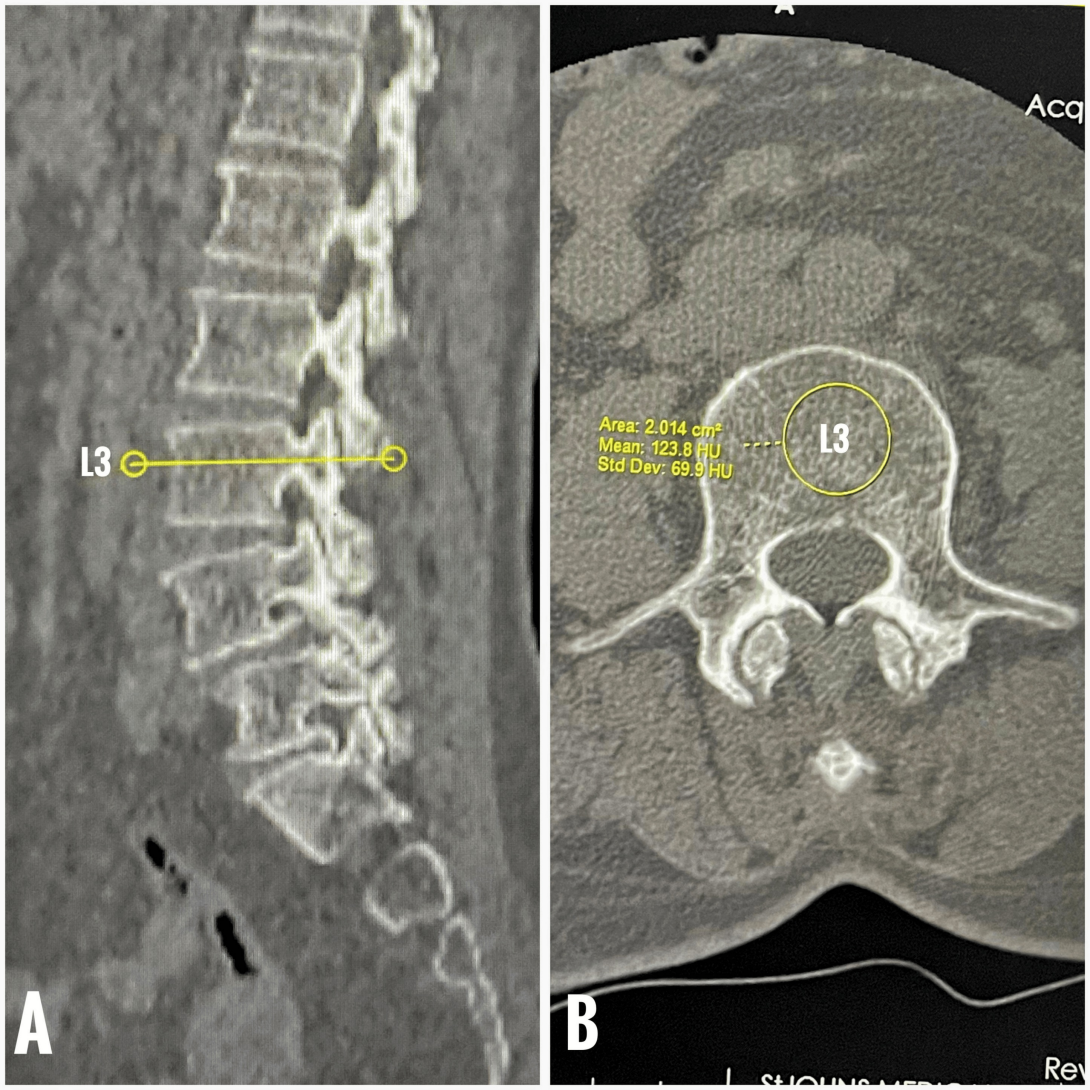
Opportunistic quantification of vertebral trabecular density using CT-derived Hounsfield unit (HU) analysis at mid-vertebral level L3.
